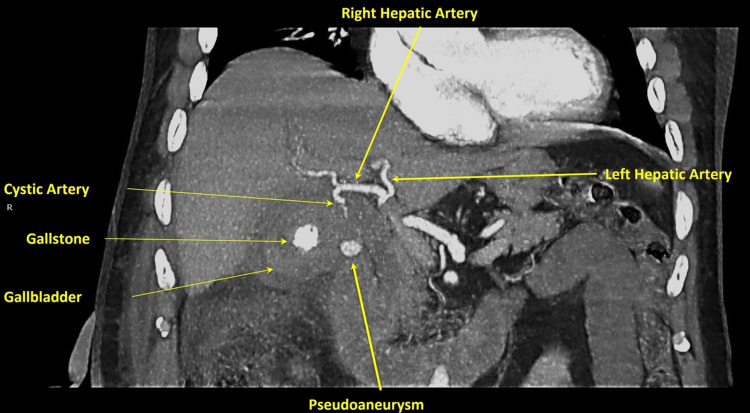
Coronal CT angiography reconstruction indicates a pseudoaneurysm arising from the cystic artery.
